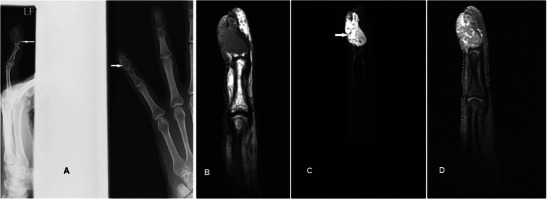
Low-grade chondrosarcoma in a 47-year-old female presenting with a slowly enlarging firm swelling over the distal phalanx of the left little finger for several months with nail deformity. (a) Plain radiograph shows an expansile lytic bony lesion in the distal phalanx of the little finger with marked endosteal scalloping and thinning with disruption of the dorsal cortex. Ring-and-arc densities are suggestive of chondroid matrix (arrows). (b) T1w sequence shows an expansile bony lesion in the little finger distal phalanx of intermediate signal, with a soft tissue component destroying and breaking through the dorsal cortex to involve the skin and nailbed. (c) The lesion is very hyperintense on T2w-FS sequence with hypointense areas (arrow), typical of a chondroid matrix. (d) Post-contrast there is heterogeneous enhancement
